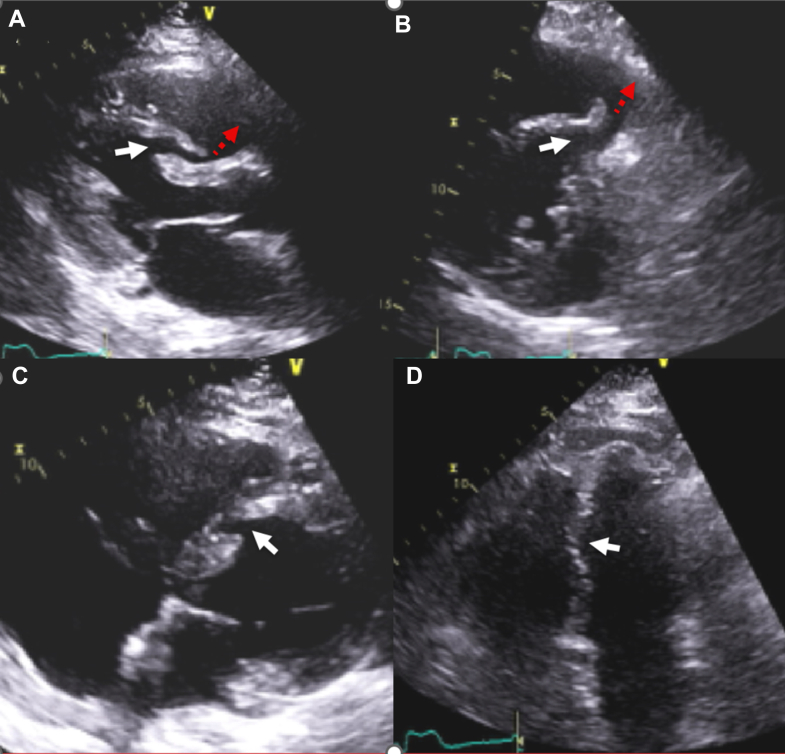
Postintervention TTE Showing Mid Cavity VSD (A and B) Parasternal long-axis and short-axis views show midcavity VSD (white arrow) dissection through the septum toward the RV (red arrows). (C and D) Four-chamber views show midcavity VSD inlet (white arrows). RV = right ventricle; TTE = transthoracic echocardiography; VSD = ventricular septal defect.
